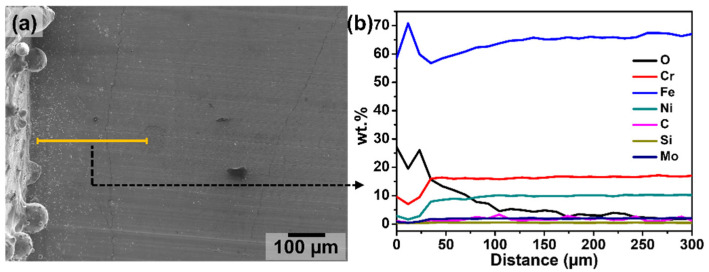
(a) Secondary electron image of a region around the HAZ, and (b) energy dispersive X-ray (EDX) composition analysis of a line scanned across the HAZ.
